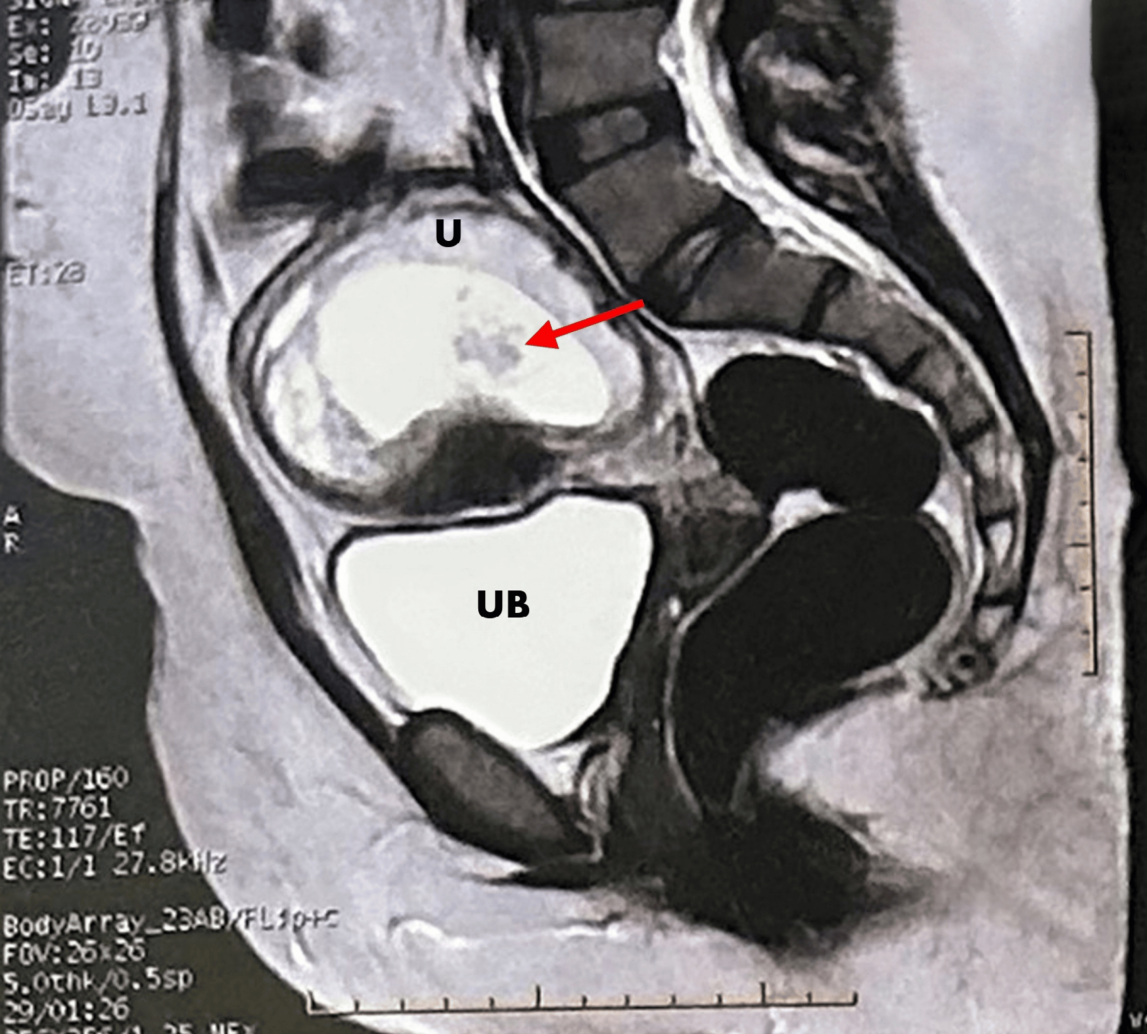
T2-weighted MRI scan T2-weighted MRI scan showing a completely adherent molar-like lesion (multi-cystic appearance) encasing the fetal sac with foci of intra-lesional blood signal inside measuring about 4.8*3*5.8 cm, thin rim of subchorionic hematoma 3 mm with no extrauterine extension and no pathological pelvic lymph nodes. MRI: magnetic resonance imaging, UB: urinary bladder, U: uterus
